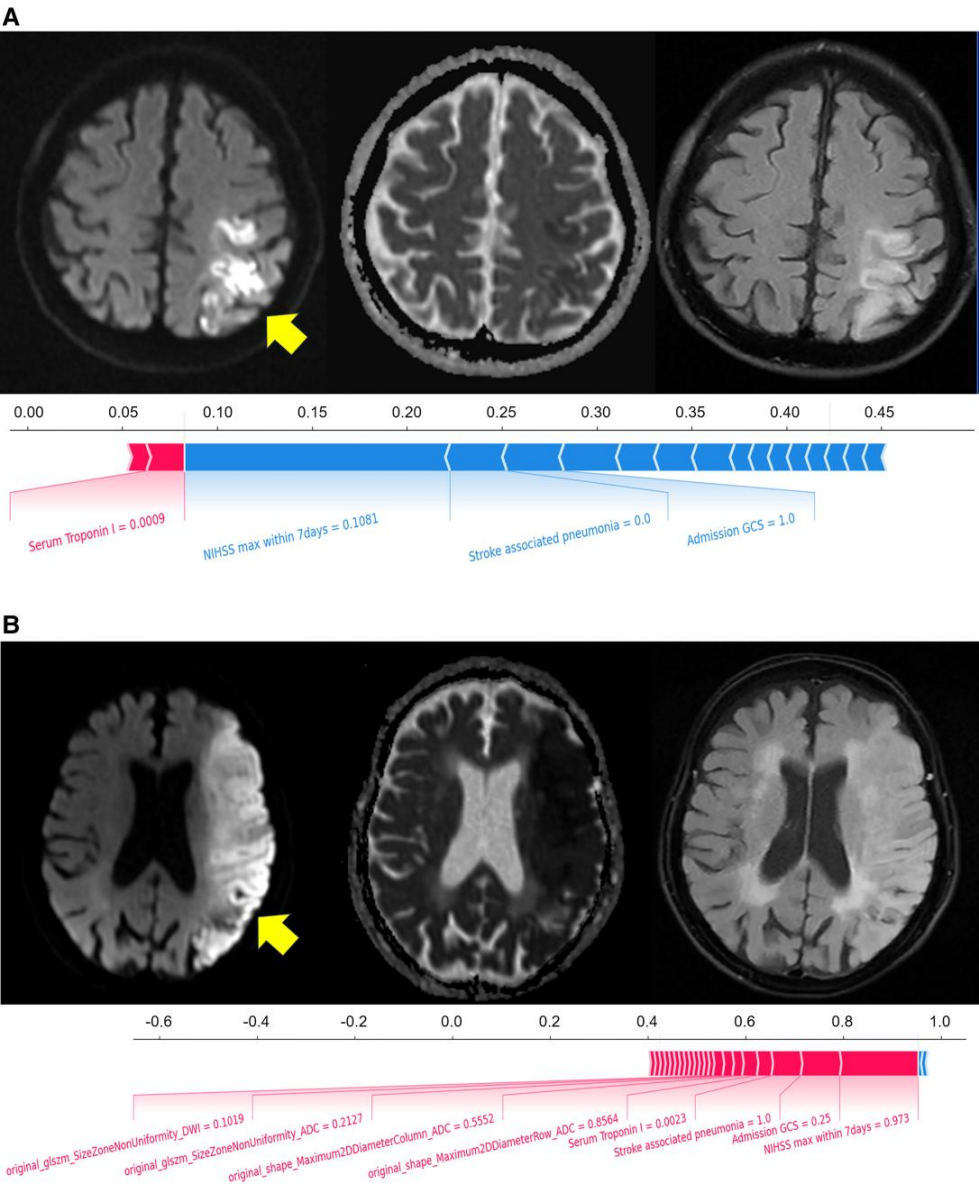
SHAP force plots of the AACNLI patients with good and poor outcome. (A) A 71-year-old male patient with 7-d NIHSS_max_ of 5- and 3-month mRS score of 2 showed a patchy AIS in the left parietal lobe on DWI (arrow), with ADC_mean_ of 523 × 10^−6^ mm^2^/s and high signal on FLAIR fat-suppression image. (B) A 68-year-old female patient with 7-d NIHSS_max_ of 17- and 3-month mRS score of 5 showed large AIS in the left temporal and occipital lobe on DWI (arrow), with ADC_mean_ of 478 × 10^−6^ mm^2^/s and slightly high signal on FLAIR fat-suppression image. The SHAP force plots of both patients show that 7-d NIHSS_max_, admission GCS score and SAP are weighted higher than other features influencing the prognosis. glszm, grey-level size zone matrix.
